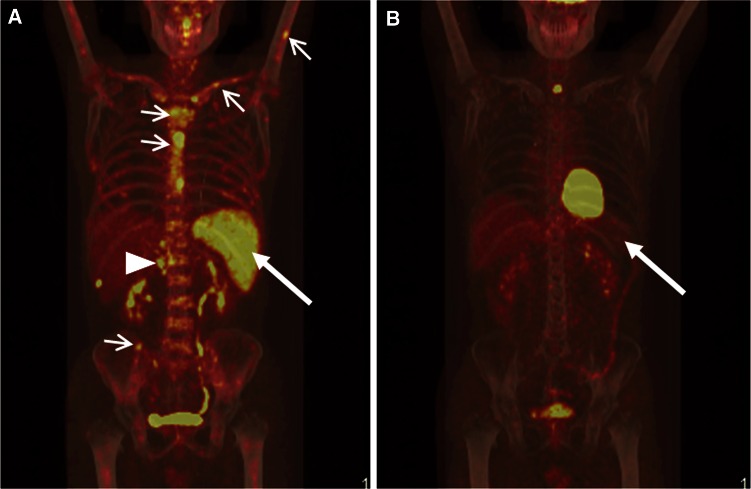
Comparison of PET–CT images of patient no. 9 before treatment and after lymphoma-chemotherapy. a Pre-therapeutic coronal PET–CT showed multiple FDG-avid lymph nodes around abdominal aorta (*triangle*), diffuse FDG uptake within enlarged spleen (*arrow*), and patchy FDG-avid lesions in sternum, clavicles, humerus, iliac bones, and multiple vertebral bodies (*arrowhead*). b After administered six cycles of DA-EPOCH chemotherapy, PET–CT revealed resolution of the metabolically active disease in multiple lymph nodes, spleen, and multiple bones, suggesting favorable efficacy of the previous chemotherapies. Until the date of cutoff time, the patient has survived for 264 days
